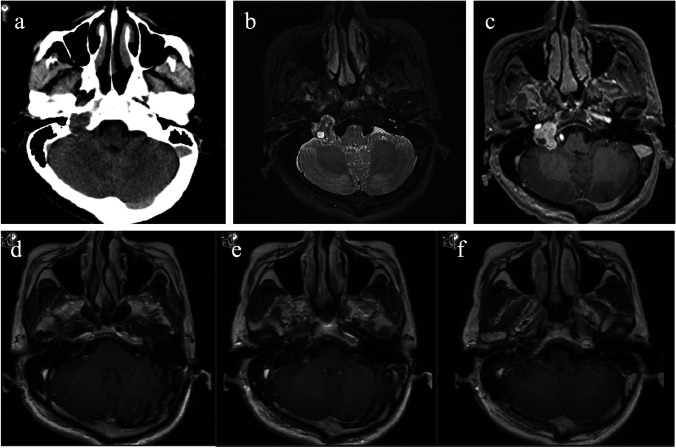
Pre-operative and post-operative radiological images. a Pre-operative CT shows a iso-density lesion located in the right jugular foramen region. b and c MRI indicates the lesion is iso-signal in T2-weighted image, and homogenous enhancement after contrast, a Samii type B schwannoma is highly suspected. d–f Post-operative MR confirms total resection of the tumor
